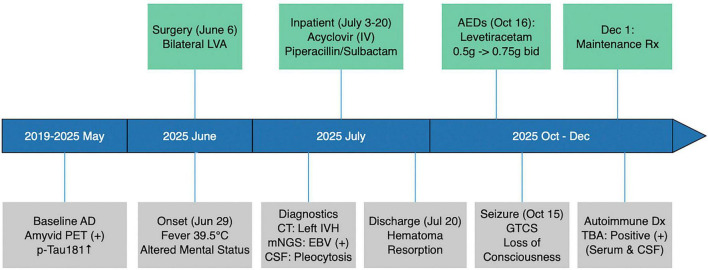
Overview of the patient’s clinical course, key diagnostic and therapeutic events, and outcomes. AD, Alzheimer’s disease; AEDs, antiepileptic drugs; bid, twice daily; CSF, cerebrospinal fluid; CT, computed tomography; EBV, Epstein–Barr virus; GTCS, generalized tonic–clonic seizure; IV, intravenous; IVH, intraventricular hemorrhage; LVA, lymphovenous anastomosis; mNGS, metagenomic next-generation sequencing; POD, postoperative day; Rx, treatment; TBA, tissue-based assay; Tmax, maximum body temperature.
